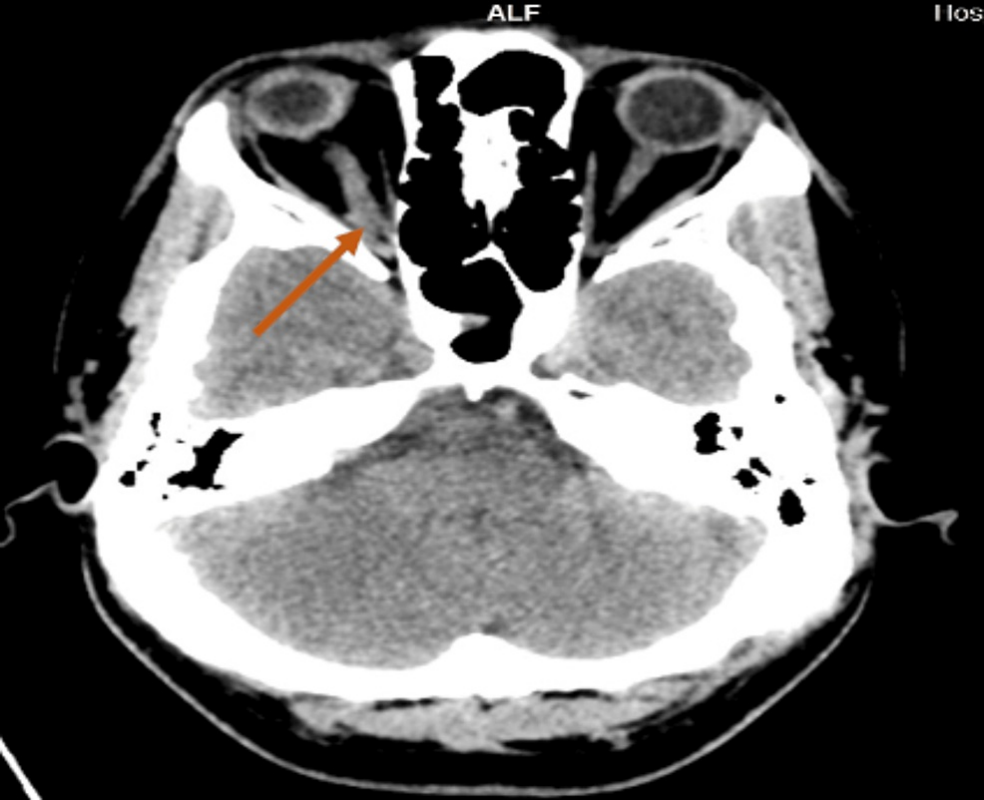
Contrast-enhanced computed tomography of the brain and orbit (axial view). Arrow: Contrast-enhanced computed tomography of the brain and orbit revealed a right unilateral enlargement of the right intraorbital and intracanalicular segments of the optic nerve, with orbital apex crowding compared to the left optic nerve. The right optic nerve demonstrated heterogeneous enhancement with surrounding fat stranding.
